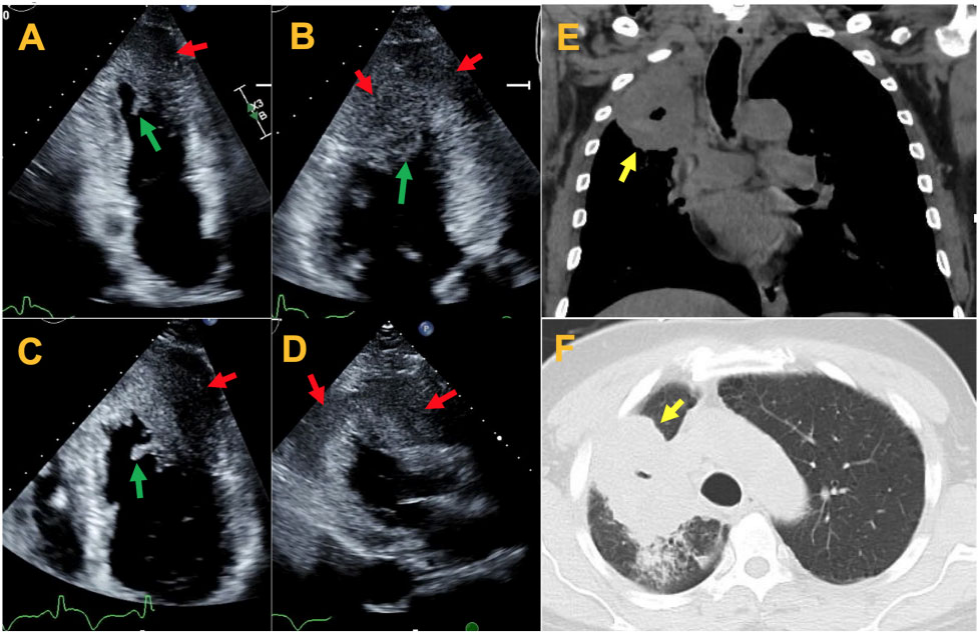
(*A–D*) Transthoracic echocardiography showing thickened wall in different views with heterogenous echogenicity (red arrows) and mobile left ventricular mural thrombi (green arrows). (*E*, *F*) Computed tomography (CT) imaging showing cavitated right upper lobe mass (yellow arrows).
